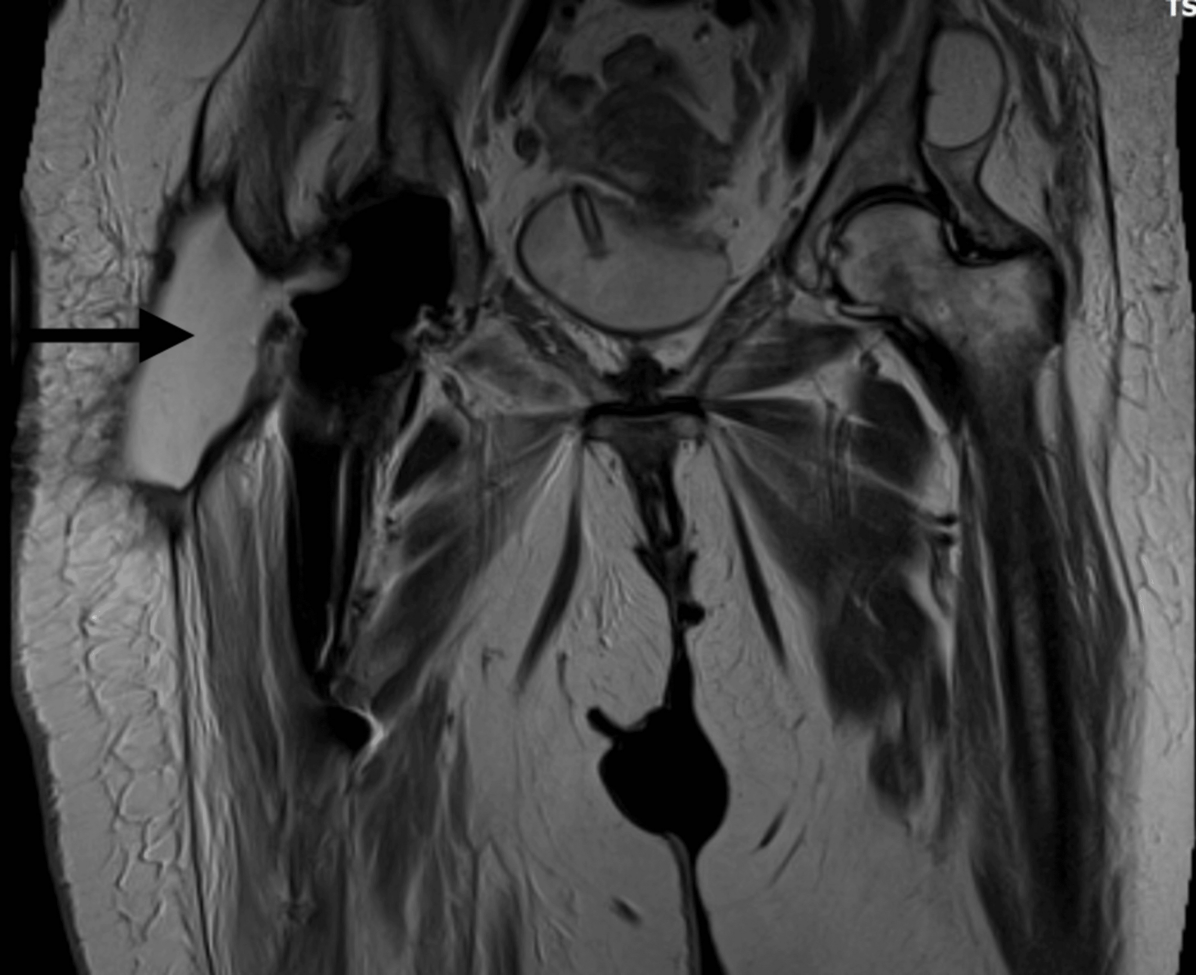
MRI demonstrating persistent periprosthetic fluid collection MRI: magnetic resonance imaging
